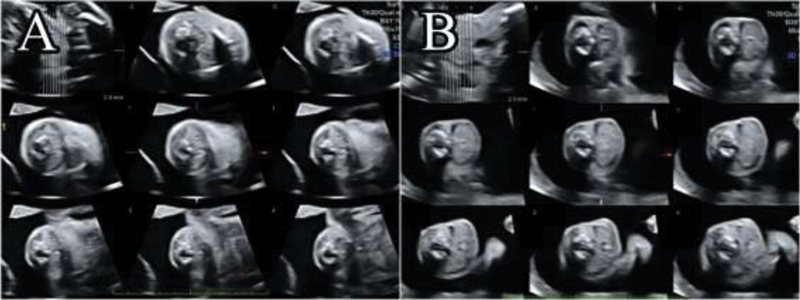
Three-dimensional ultrasound in the tomographic ultrasound imaging mode of the thyroid gland of fetus 1 (
